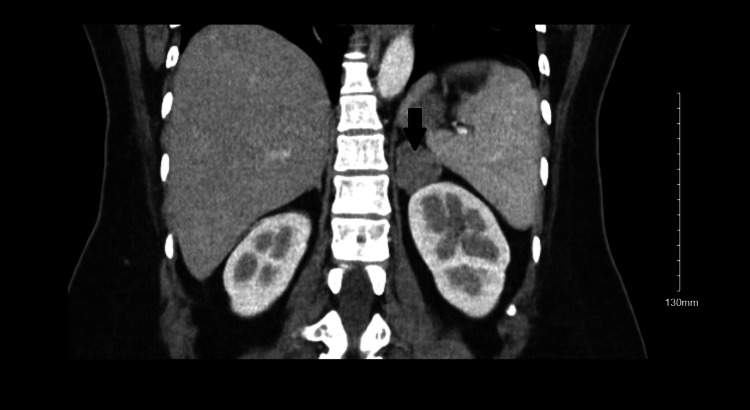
CT abdomen pelvis (coronal view) showing left-sided adrenal adenoma
